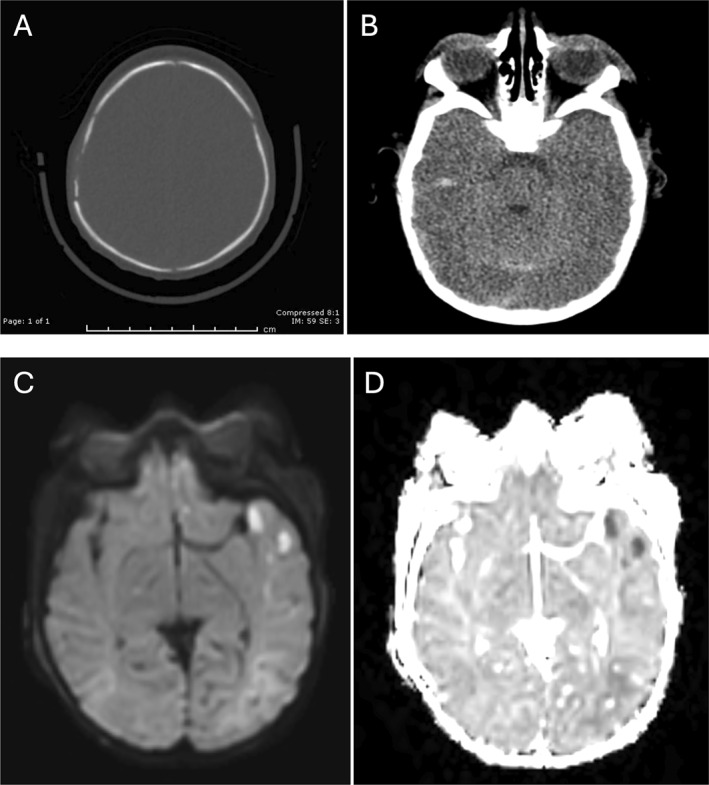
A 5‐week‐old male presented with no known history of trauma who presented during the pandemic with seizure activity and was found to be a victim of unexplained polytrauma, including: (A and B) CT images demonstrating complex right parietal bone fracture, multicompartmental hemorrhage, and cerebral edema and (C and D) MRI demonstrates extensive intracranial injury with multiple areas of restricted diffusion reflecting a combination of contusions, diffuse axonal injury, and watershed infarcts.
